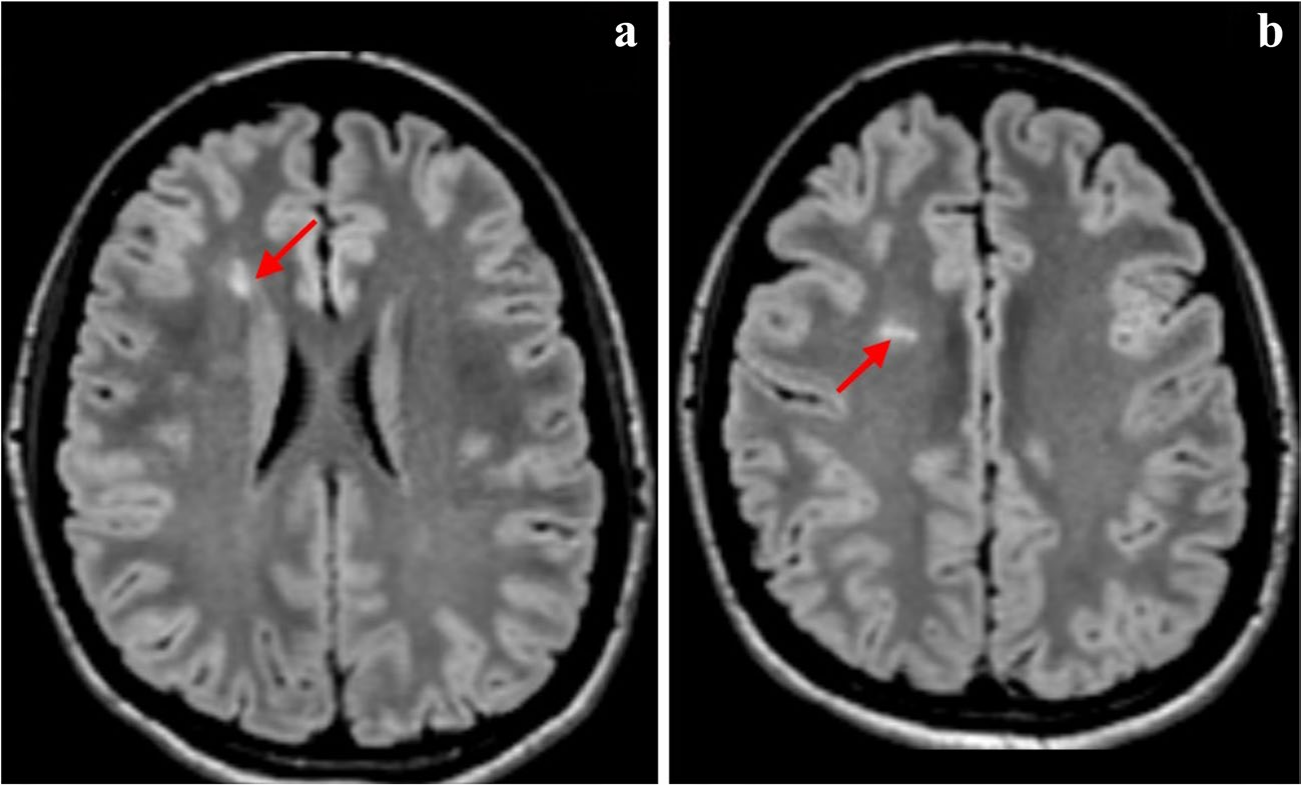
MRI of the patient 4.0. Discrete pathological MRI involvements. Axial FLAIR shows two small lesion in the periventricular white matter (a, b)
